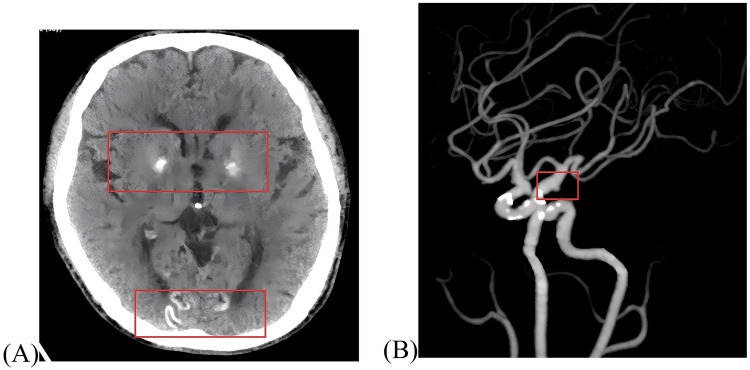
Head and neck computed tomography angiography (CTA) in the patient with Fabry disease. (A) Symmetrical intracranial calcifications involving the bilateral cerebellar hemispheres, basal ganglia, and occipital lobes. (B) A suspected intracranial aneurysm in the C7 segment of the right internal carotid artery.
